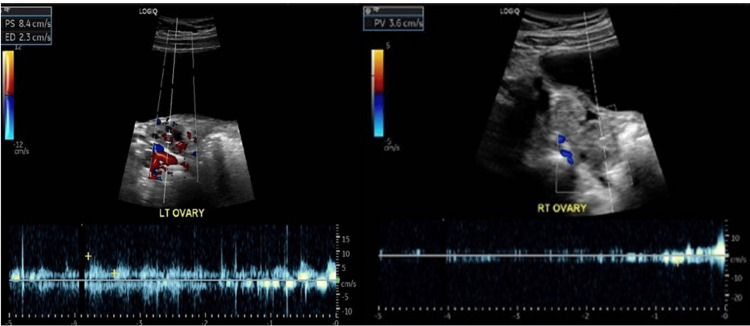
Doppler ultrasound of patient's ovaries The images demonstrate normal blood flow to the patient's left ovary (left) compared to the restricted blood flow to the torsed right ovary (right)
